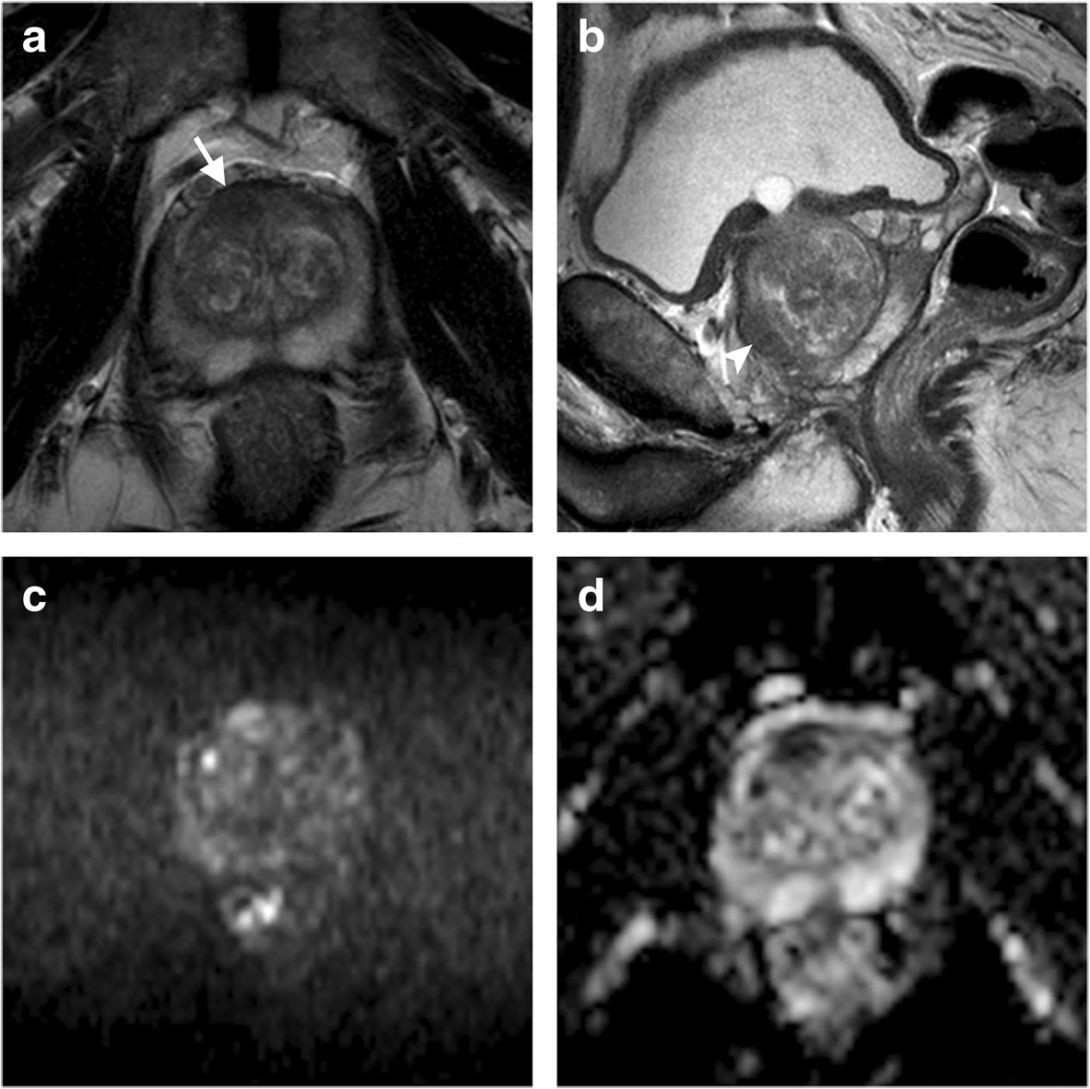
Use of PI-RADS version 2 criteria to categorise a transition zone (TZ) finding in a 67-year-old patient with elevated PSA (7.80 ng/mL) and previous negative biopsies. On transverse (arrow in a) and sagittal (arrowhead in b) T2-weighted images, there was an anterior, right-sided TZ focal area with lenticular shape, ill-defined margins, and capsular bulging (< 15 mm in size), close to the anterior fibromuscular stroma. This finding was scored as PI-RADS 4 and confirmed to be a Gleason score 6 (3 + 3) cancer on biopsy and subsequent radical prostatectomy (T2b N0). DWI supported the suspicion of malignancy by showing restricted diffusion as corresponding, focal hyperintensity on *b* = 2000 s/mm^2^ image (c) and marked hypointensity on the ADC map (d)
